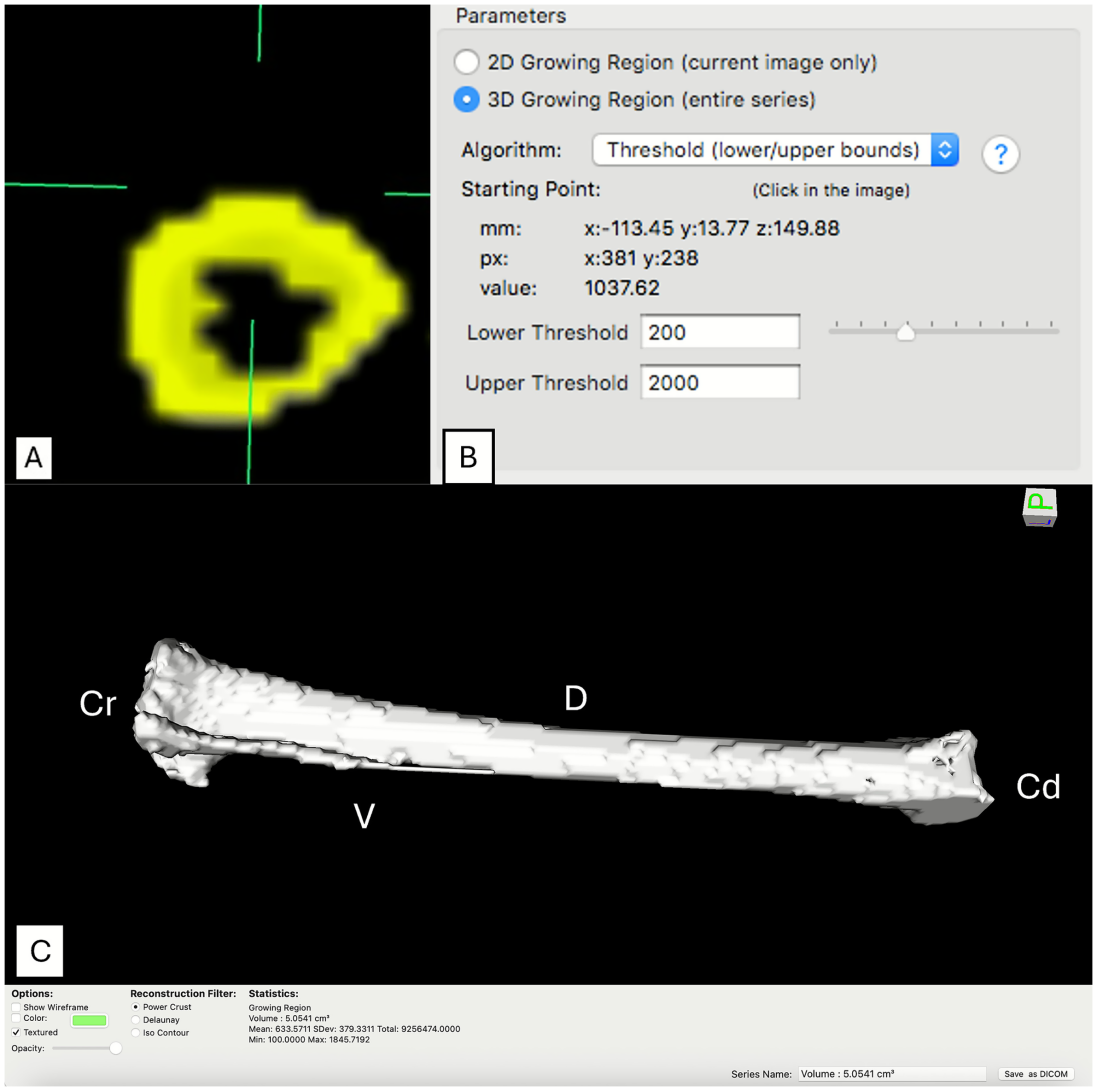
Segmentation procedure and cortical analysis by computed tomography. (A) Example of Region Growing segmentation using the “2D Grow Region” tool in a transverse tibial slice, in which the entire mineralized bone area (highlighted in yellow) was selected for evaluation; (B) definition of radiodensity threshold parameters (200 to 2000 BMD) for selective inclusion of cortical bone tissue; (C) three-dimensional reconstruction of the right tibia of laying hens obtained by computed tomography (CT). Anatomical orientation is indicated (Cr, cranial; Cd, caudal; D, dorsal; V, ventral).
